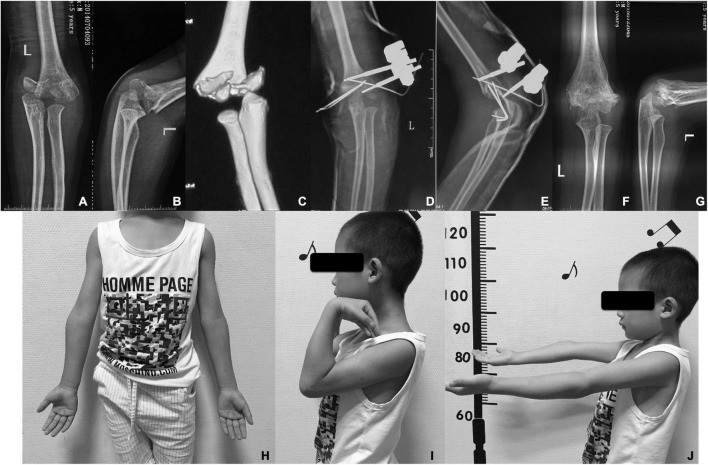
(A) Anteroposterior and (B) lateral radiographs of a 6-year-old boy with an intercondylar fracture of the distal humerus; (C) CT scan showing C1 type of AO classification; (D) anteroposterior and (E) lateral radiographs post-operation; (F) anteroposterior and (G) lateral radiographs at 12 months post-operation; and the follow-up in 24 months after surgery show excellent cosmetic results (H) and the functional appearance (I,J).
